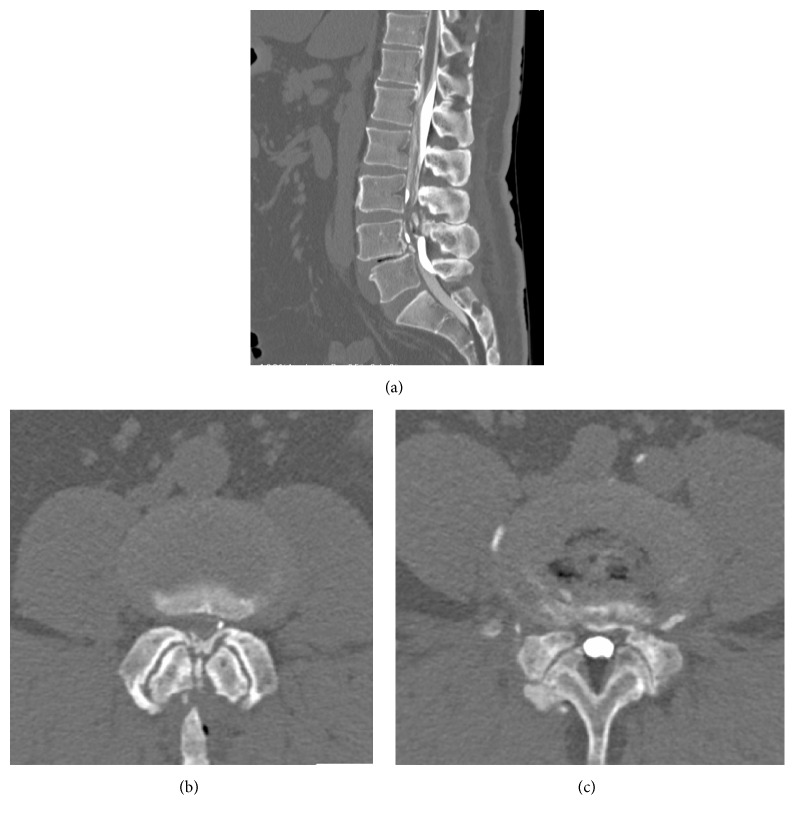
Preoperative computed tomography (CT) myelography. Sagittal image at the midspinal canal (a); axial image at the L3/4 level (b); axial image at the L4/5 level (c). OYL and complete block are seen at the L3/4 level.
